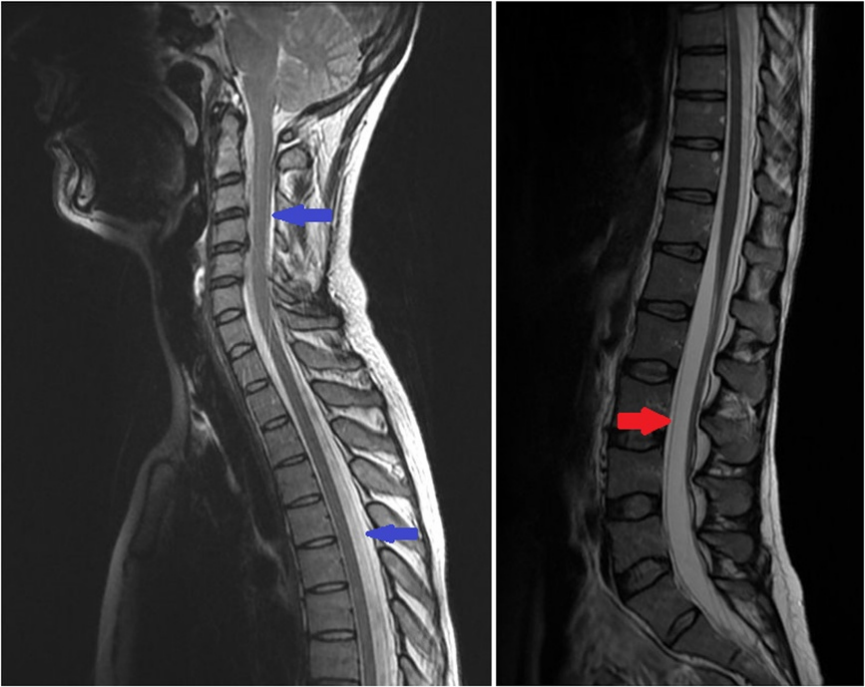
T2-weighted magnetic resonance imaging (saggital section) showing cerebrospinal fluid collections anterior to the theca at L2-S2 (red arrow) and posterior to the spinal cord at the level of T1-9 and also at C1-2 (blue arrows). There is no evidence of spinal cord compression and the spinal cord has normal signal.
